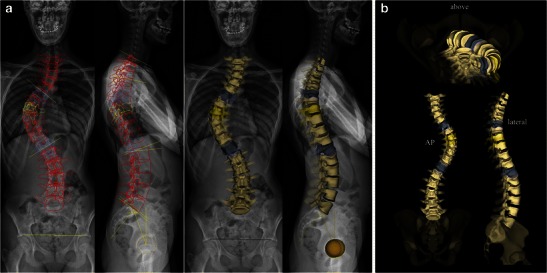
Surface 3D reconstructions of the spine in a 13-year-old girl with idiopathic thoracic scoliosis. A 3D model of the spine (indicated in *red*) is generated by the software and overlayed to the native AP and lateral radiographs (a). This model has to be manually adjusted by the operator to precisely match the spinal anatomy. Different perspectives of the spinal deformity are obtained, showing the right thoracic curvature, superior (T6) and inferior (T12) end vertebrae (in *blue*), and the apical vertebra (T8) in *yellow* (b). Measurements based on the 3D model are finally automatically computed, including values for spinal curvatures, axial vertebral rotation and pelvic parameters. [Total DAP = 626 mGy·cm^2^]
